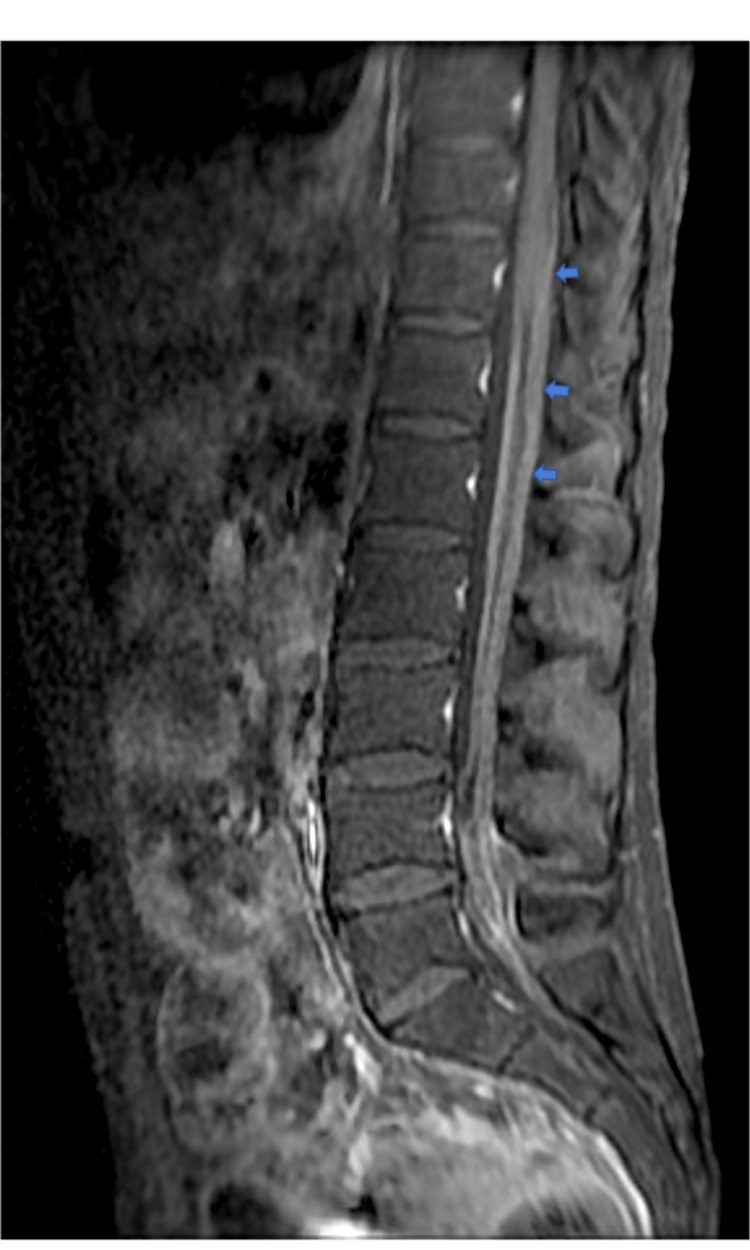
Post-contrast T1-weighted MRI image in sagittal plane demonstrating diffuse leptomeningeal enhancement (shown by arrows) at the time of presentation
